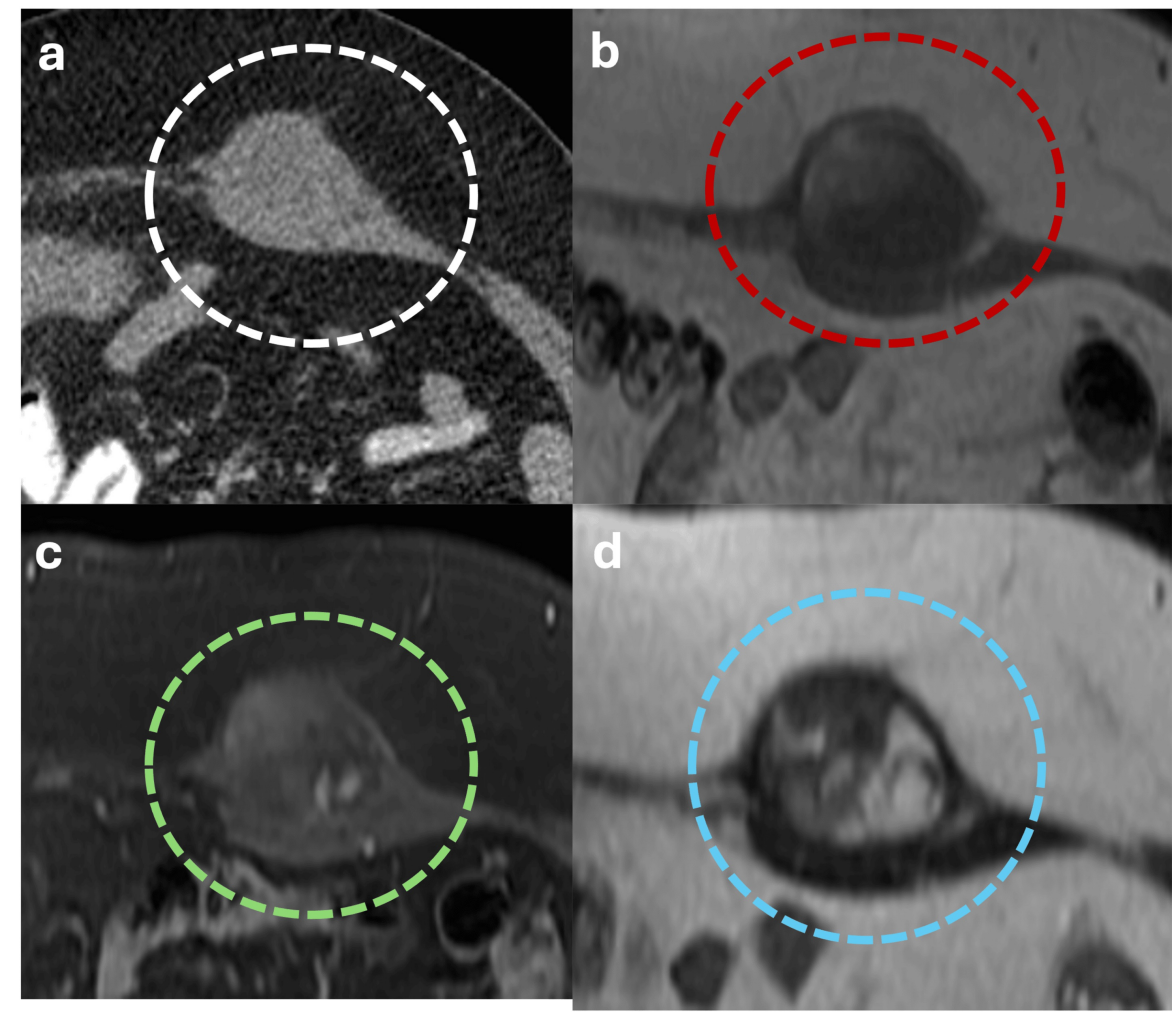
Axial contrast-enhanced abdominal CT and MRI showing a desmoid tumor (a) Axial CT reveals a soft-tissue density lesion with heterogeneous content and regular borders in the anterior abdominal wall (white dotted circle). (b, c) Axial MRI showing the same lesion depicting a T1 hypointense signal with poor contrast enhancement after contrast medium administration (red and green dotted circles, respectively); (d) the lesion exhibits a T2 hypointense signal with focal areas of high signal intensity due to cellular myxoid content (blue dotted circle), these findings collectively are consistent with desmoid tumor. The figure was created by the authors.
